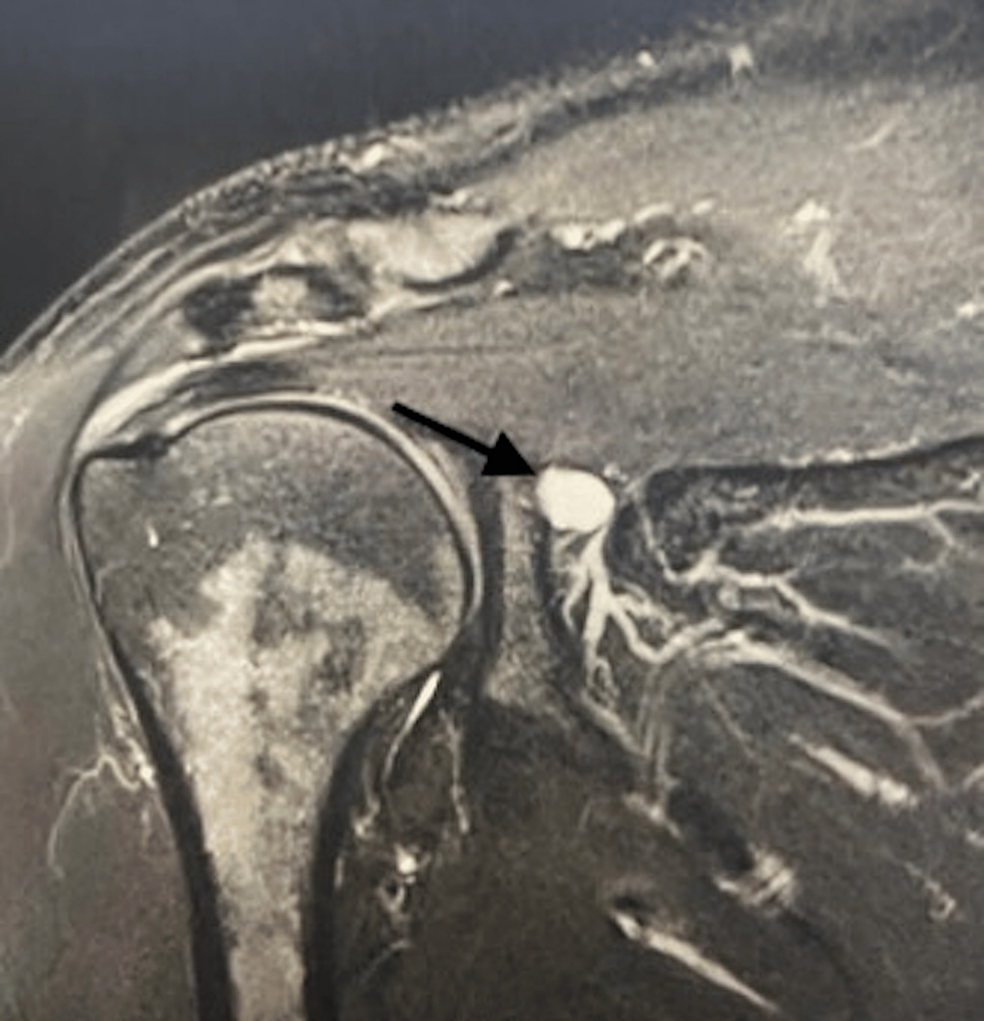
Coronal MRI scan of the left shoulder showing the spinoglenoid notch ganglion cyst
